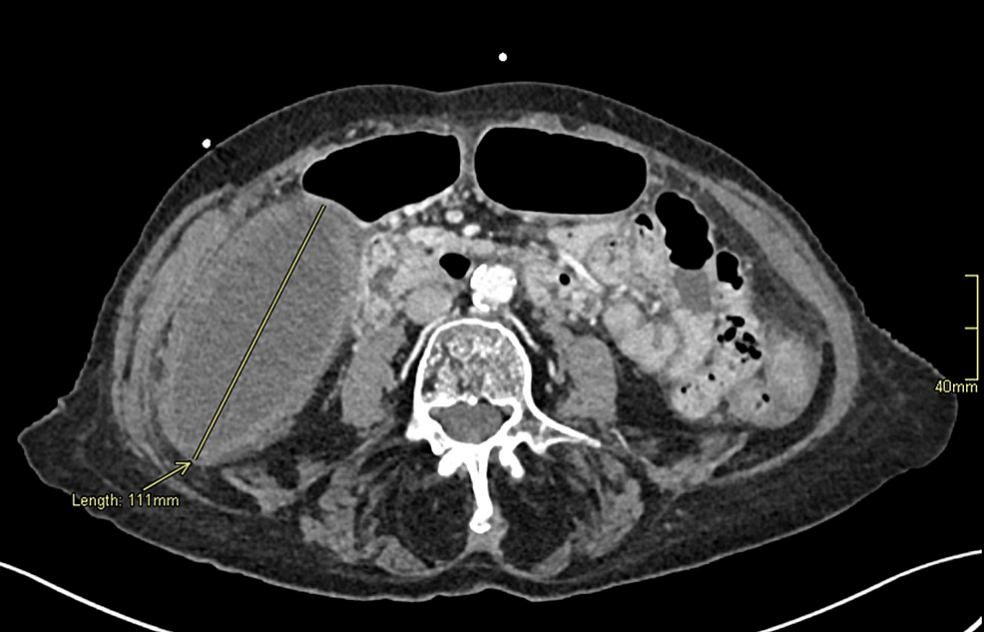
Axial contrast-enhanced computed tomography of the abdomen and pelvis Yellow arrow and line show a thick-walled, distended gallbladder measuring 11.1 cm in the right abdominal cavity
